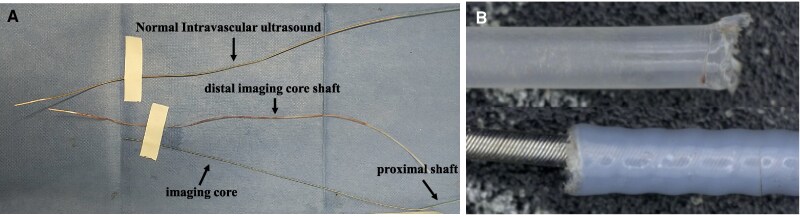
The broken intravascular ultrasound catheter. (*A*) The picture shows the normal (upper) and fractured (lower) intravascular ultrasound shafts. (*B*) A magnified picture of the broken site.
